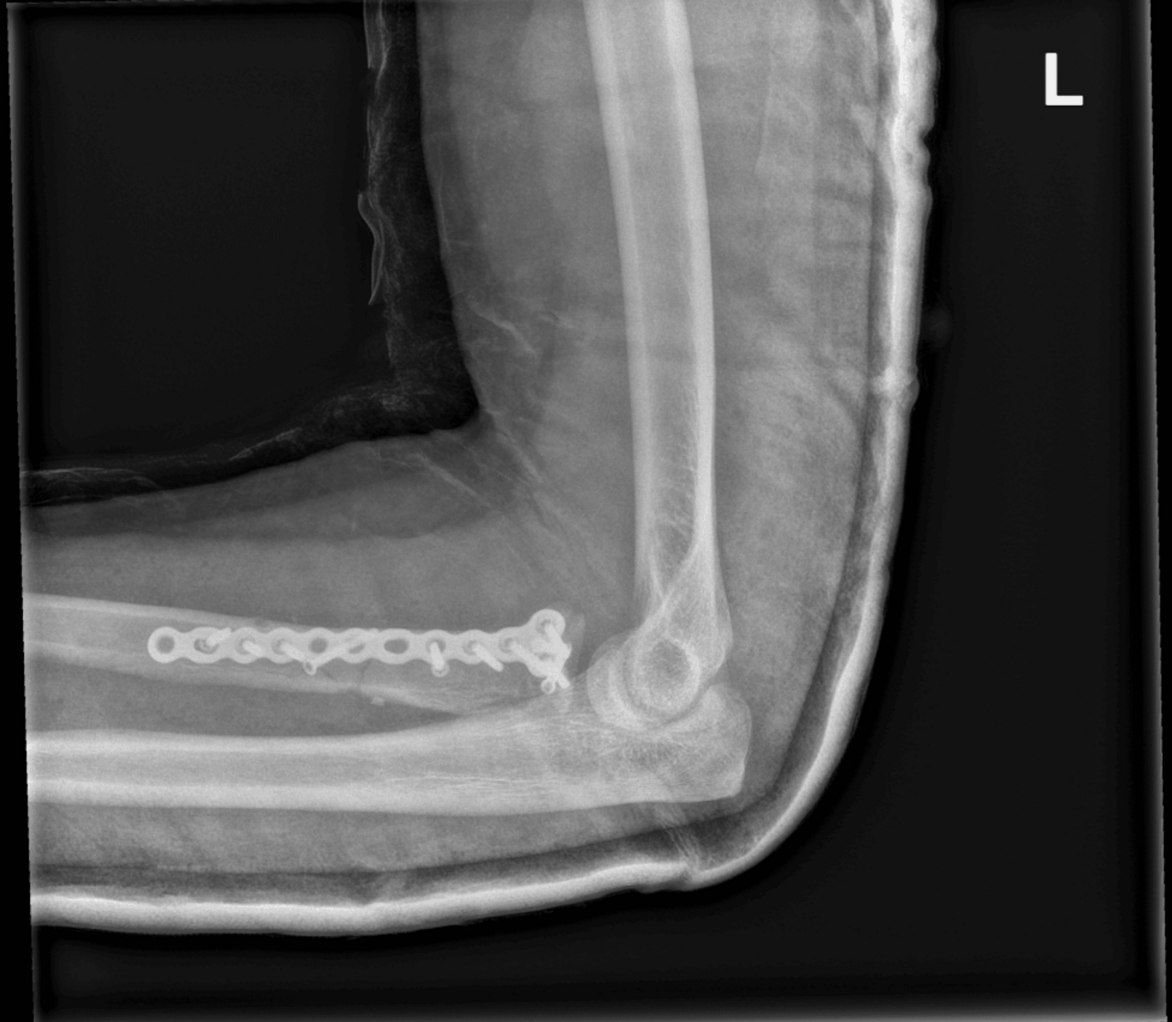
Lateral view of postoperative radiograph at two weeks
